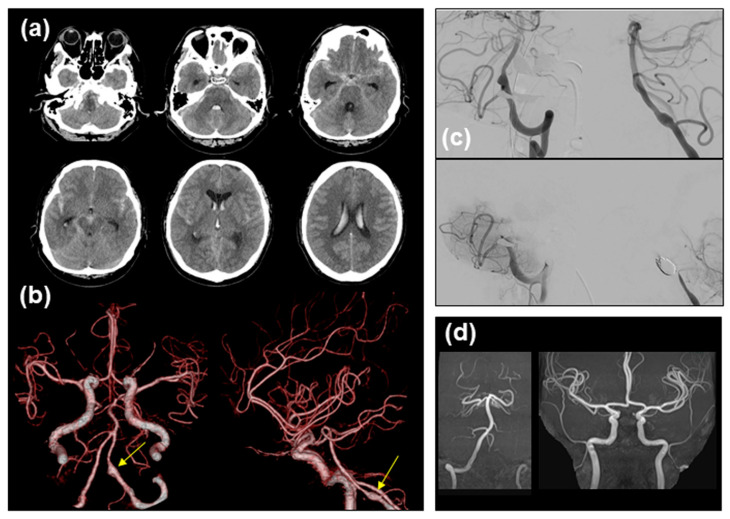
Imaging findings. Initial brain CT scan (a) and CT angiography ((b) (left), anteroposterior projection; (right), left lateral projection). A left VADA ((b) arrows) successfully treated by endovascular trapping. (c) DSA before (upper panels) and after (lower panels) the treatment. (d) MR angiography of the posterior (left panel) and anterior (right panel) circulation on day 14.
